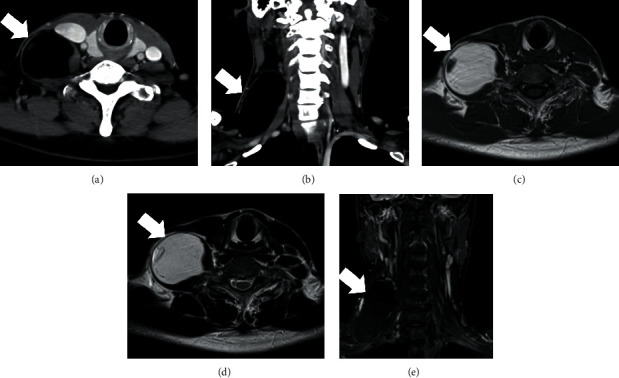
Imaging findings: contrast-enhanced computed tomography ((a) horizontal section and (b) coronal section) showing a well-defined, 85 × 50 mm tumor (arrow) in the right neck. There was no contrast effect inside the tumor. Contrast-enhanced MRI ((c) T1-weighted images, (d) T2-weighted images, and (e) STIR) shows the tumor had low signal intensity on T1-weighted images, high signal intensity on T2-weighted images, and low signal intensity on STIR. Variable signal intensity areas were seen outside the tumor.
